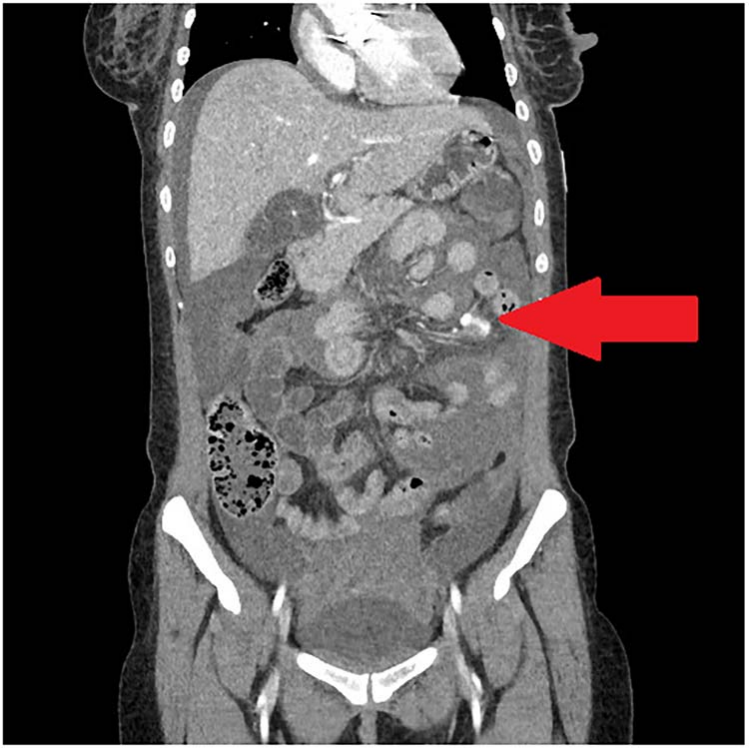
Portal-venous phase CT abdomen demonstrating haemoperitoneum and contrast extravasation in small bowel mesentery.
